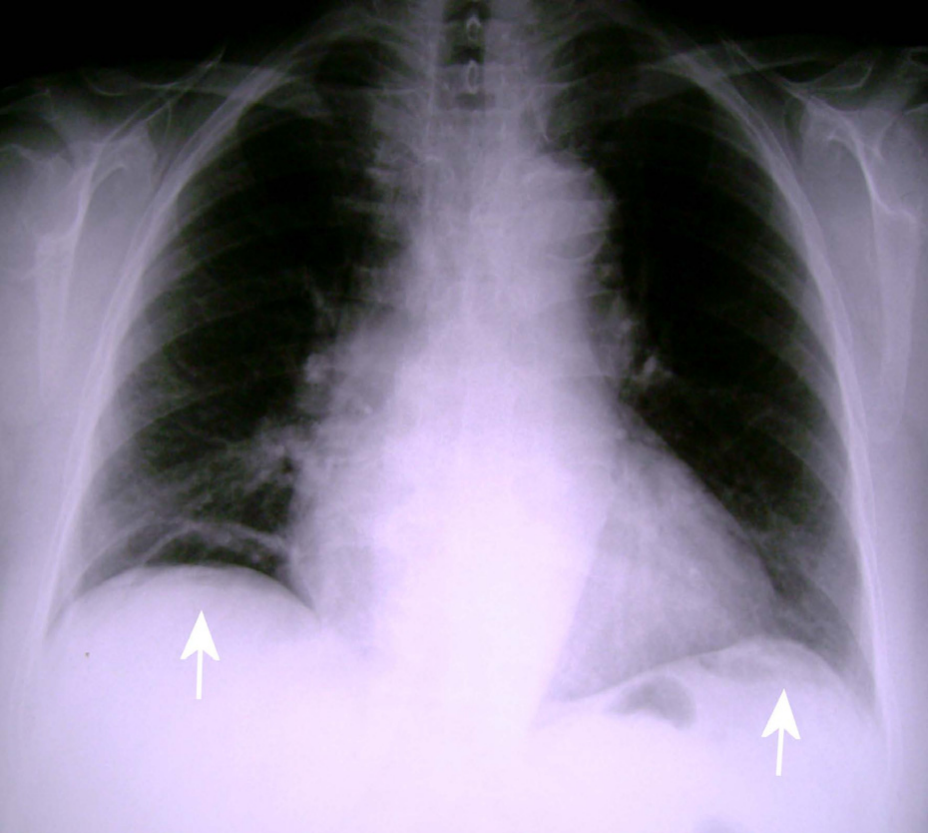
Upright posteroanterior chest radiograph. There is free subdiaphragmatic air bilaterally that is more clearly noted on the right side (*white arrows*).
